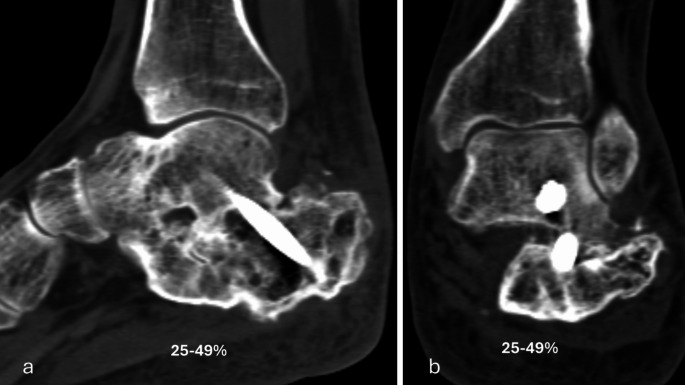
Six months lateral (a) and anteroposterior (b) CT scan of the subtalar arthrodesis showing 25–50% fusion rate
